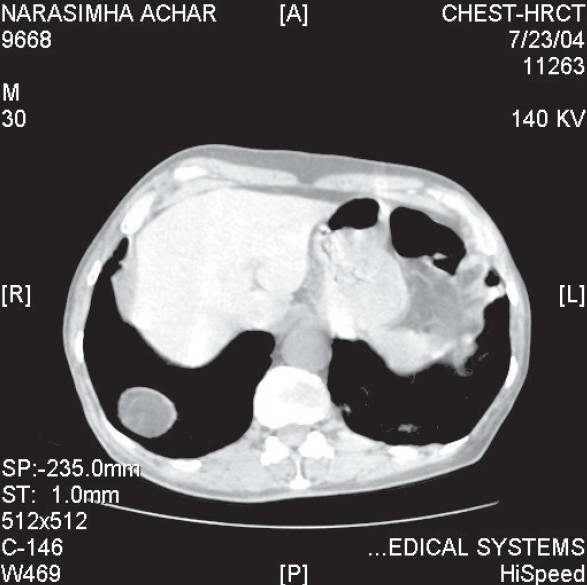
CT chest showing a right lower lobe intrapulmonary lesion of fat density.
